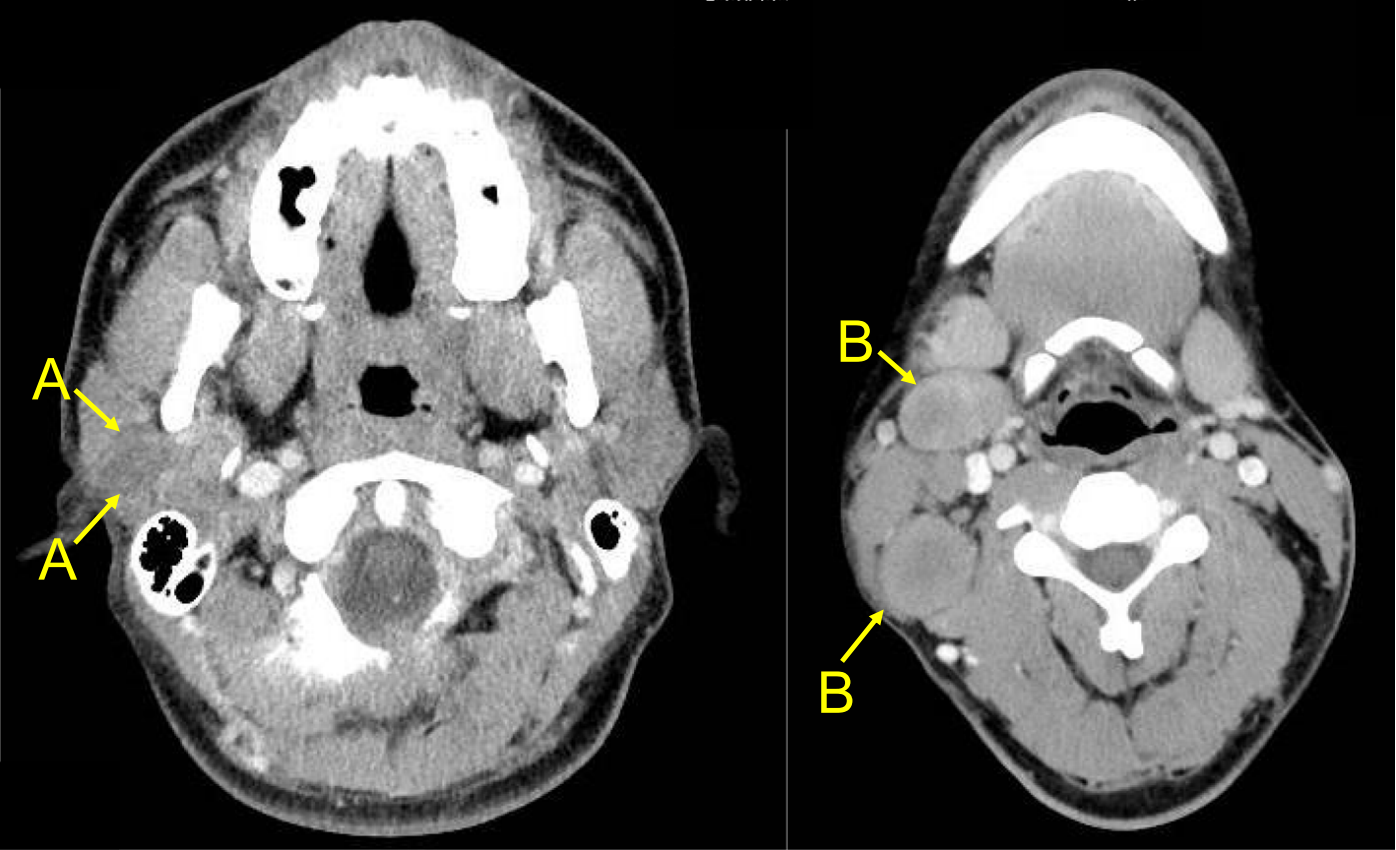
Head computed tomography shows tumor at right parotid gland (a) and lymphadenopathy of right neck (b)
